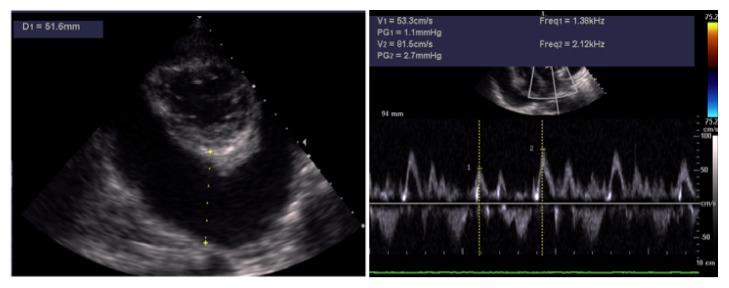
Transthoracic echocardiography*. Parasternal short axis view (apex) showing a large pericardial effusion (>5 cm) with a swinging heart. Pulse wave Doppler (PWD) examination shows significant respiratory variations (i.e., >25%) in left ventricular diastolic filling pattern.
